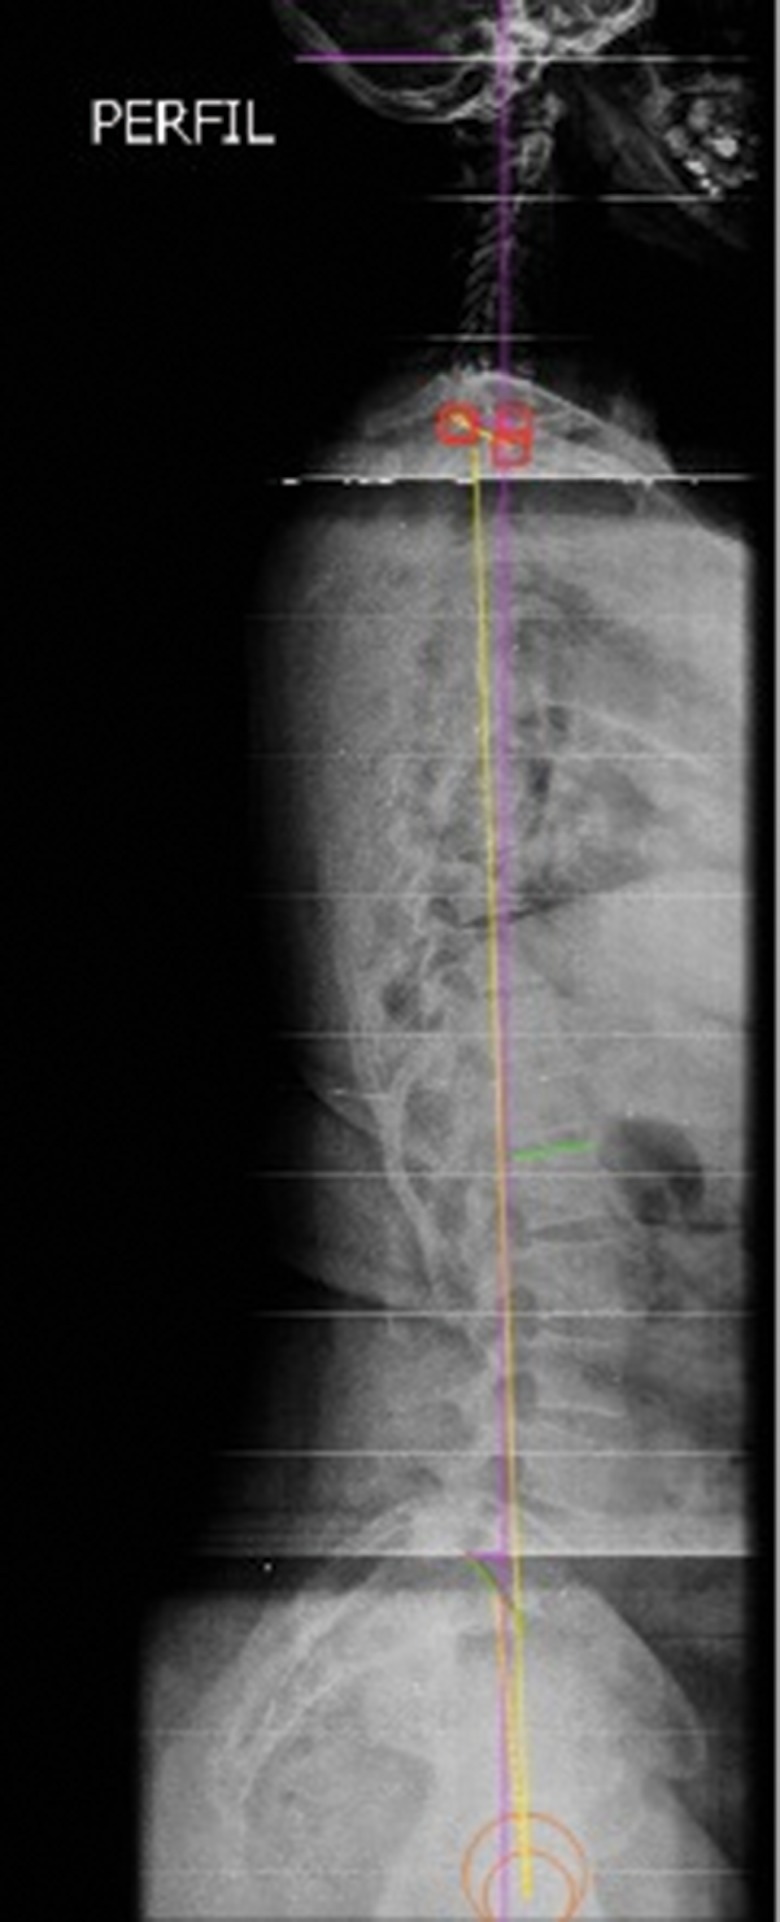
Example of measurement of the radiographic parameters using Surgimap software (Nemaris Inc. New York, USA).
